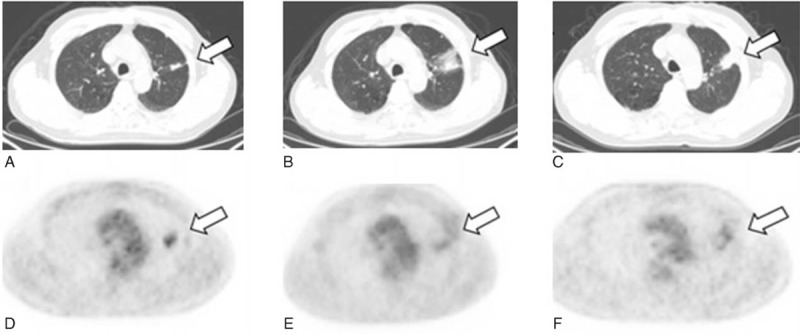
PET/CT-guided RFA applied for a 60-year-old male patient with NSCLC (squamous cell carcinoma of 1.9 × 0.7 cm in size). Split dose ^18^F-FDG methodology included pretreatment with 4 mCi of FDG followed by a postprocedure dose of 8 mCi for same-site treatment assessment. In comparison with a preoperative ^18^F-FDG PET/CT imaging (A, D), the size and metabolic state of the residual tumor were accurately characterized with split-dose ^18^F-FDG PET/CT reimaged at 45 minutes (B, E) and 3 months (C, F) after RFA respectively. The images revealed a focal ^18^F-FDG activity in the ablation zone at 45 minutes and 3 months after the treatment. ^18^F-FDG = ^18^F-2-fluoro-2-deoxy-d-glucose, NSCLC = nonsmall cell lung cancer, PET/CT =  positron emission tomography/computed tomography, RFA =  radiofrequency ablation.
